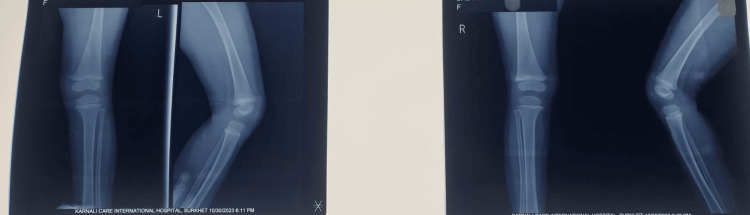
Anteroposterior and lateral radiographs of the right and left knee at two years of age
